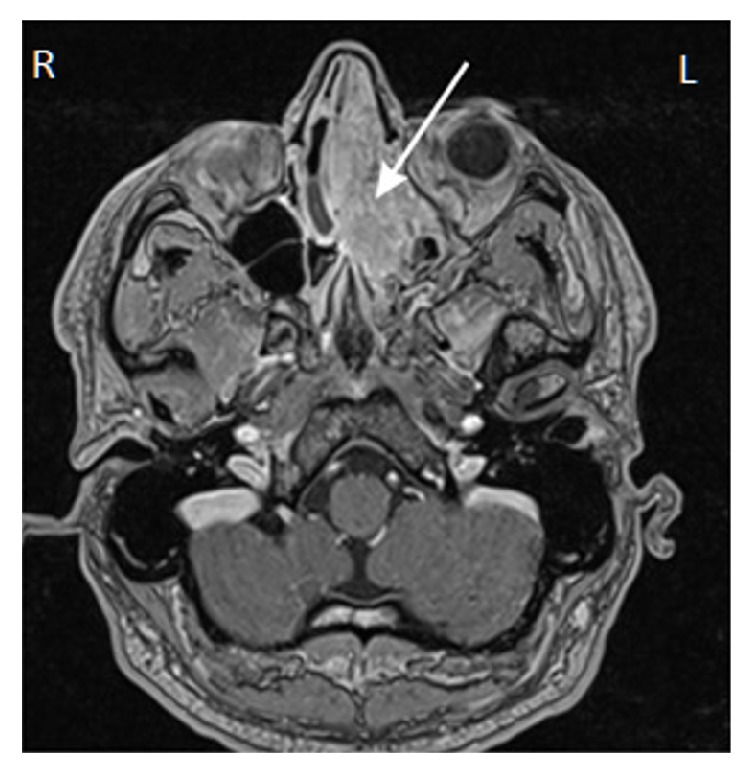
MR image along the transverse plane in a patient with left side (arrow) sinonasal inverted papilloma with visible tumor mass compression of the opposite side.
